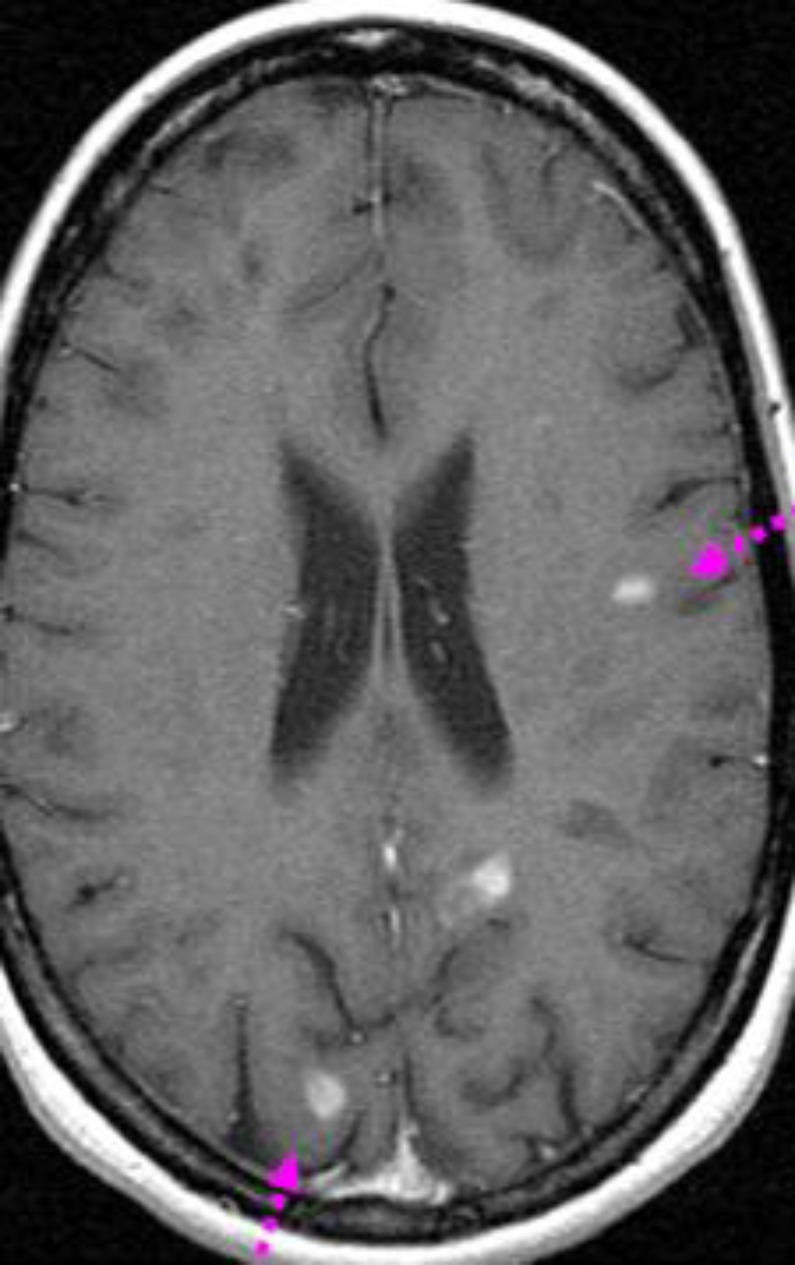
Cerebral MRI enclosed multiple T2 hyperintensities of brainstem, cerebellum, corpus collosum and periventricular white matter
